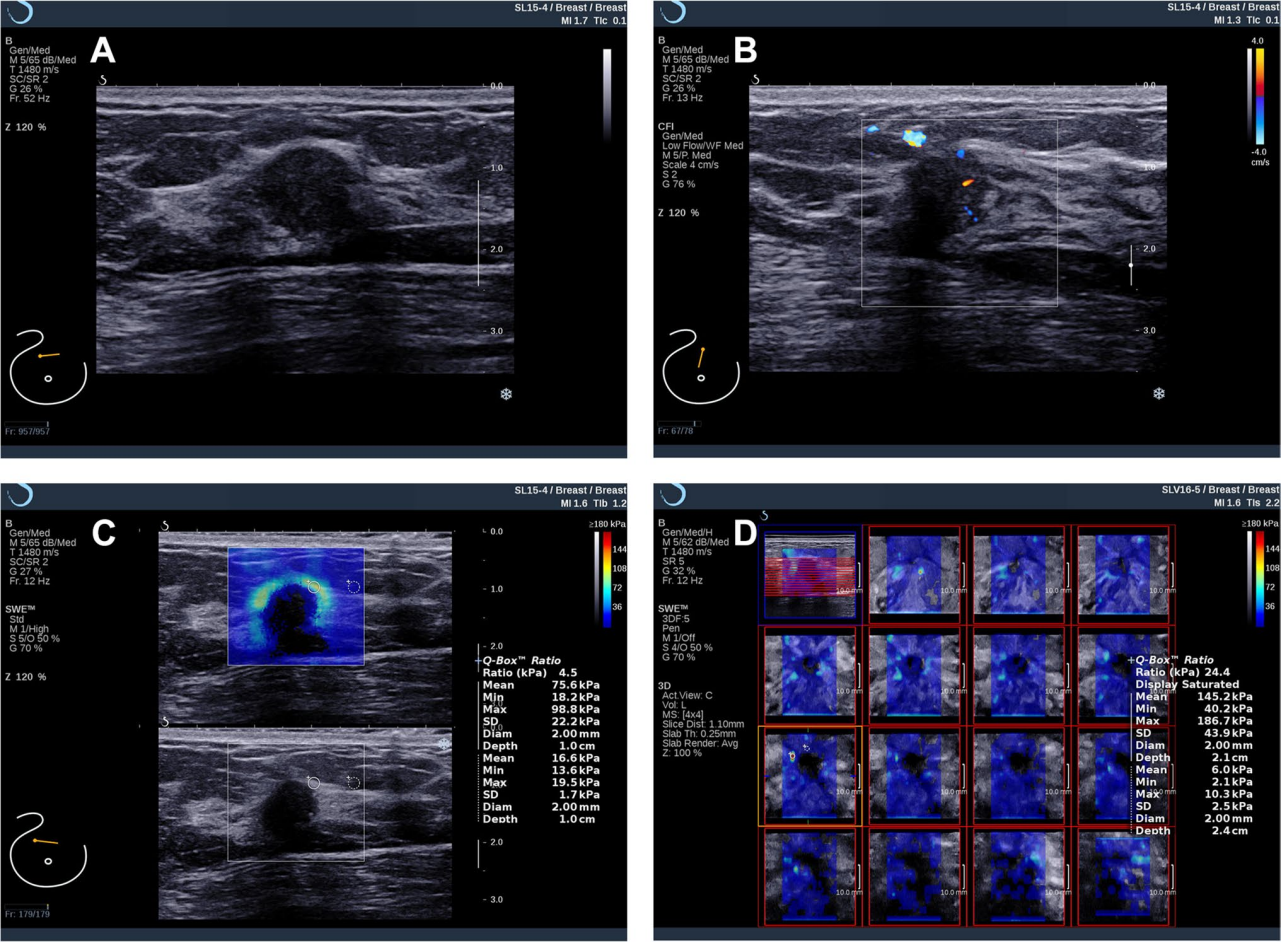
A 55-year-old female participant with papillary cancer in the right breast. BI-RADS 3 was assessed by standard breast US examination using a B-mode ultrasound image (A) and a color Doppler image (B). C A mean elasticity of 75.6 kPa in the 2D SWE only assessment indicated no change in the BI-RADS category. D A mean elasticity of 145.2 kPa in 2D + 3D SWE assessment indicated that upstaging to BI-RADS 4a should be carried out
